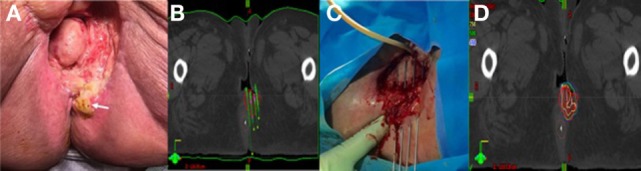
(A) Pretreatment image of Patient A’s posterior lesion adjacent to the anus (white arrow). (B) Preplanning computed tomography (CT) scan for patient A with mock-up of needle placement. (C) Treatment setup for patient A showing placement of guide needles (prior to flexible breast catheter placement). (D) Brachytherapy treatment plan for patient A (red = gross tumor, brown = rectum).
